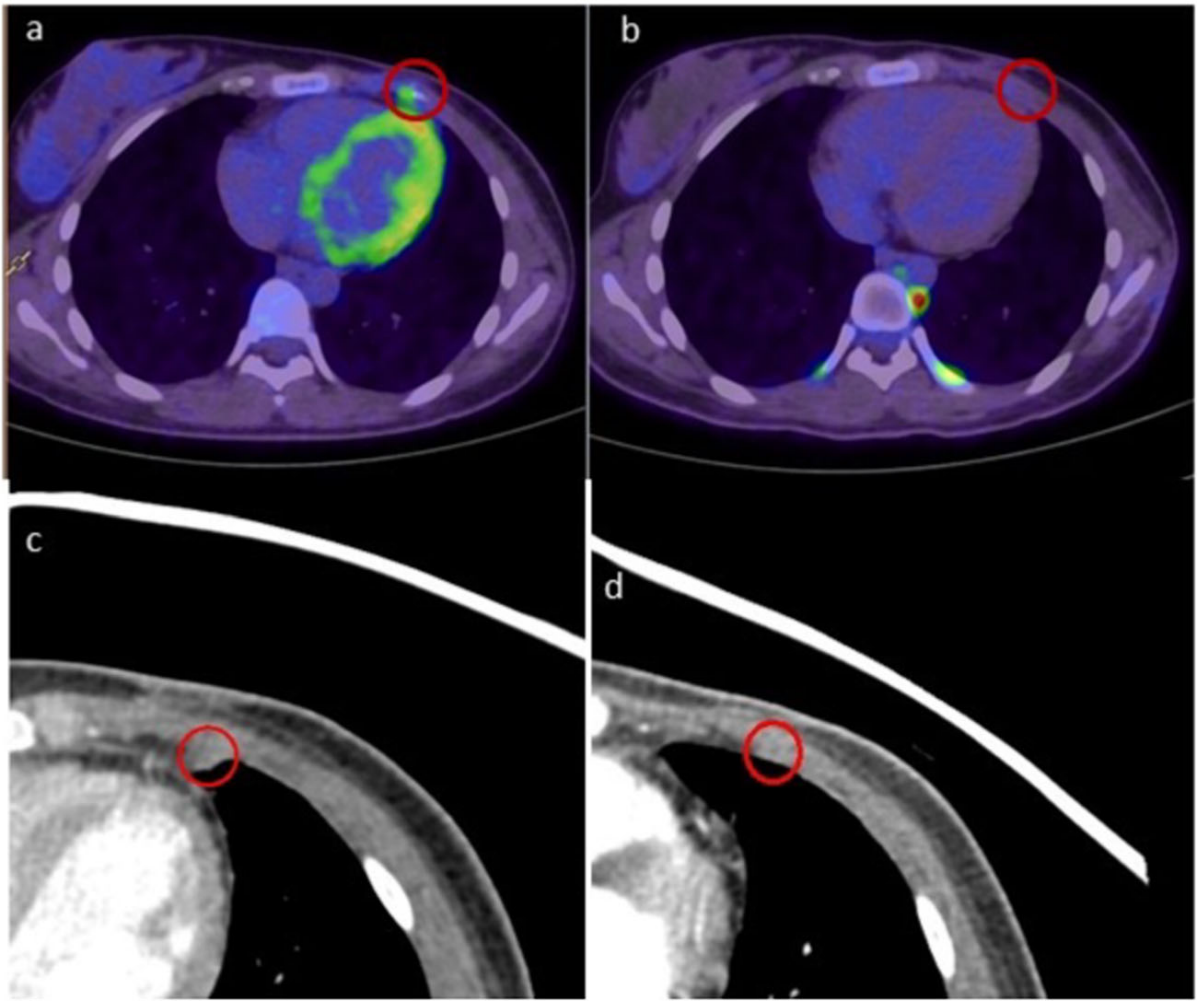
Comparative fused FDG-PET/CT images of the left 4th intercostal space nodule. a Focal avid disease (red circle) before commencing trametinib in May 2019. b Complete metabolic response after 15 months of treatment in September 2020 (with physiological brown fat activity in paravertebral and costovertebral regions). Comparative high-resolution CT images demonstrating (c) 6.5-mm lesion before commencing trametinib and (d) complete radiological response after 6 months of treatment with trametinib in October 2019.
